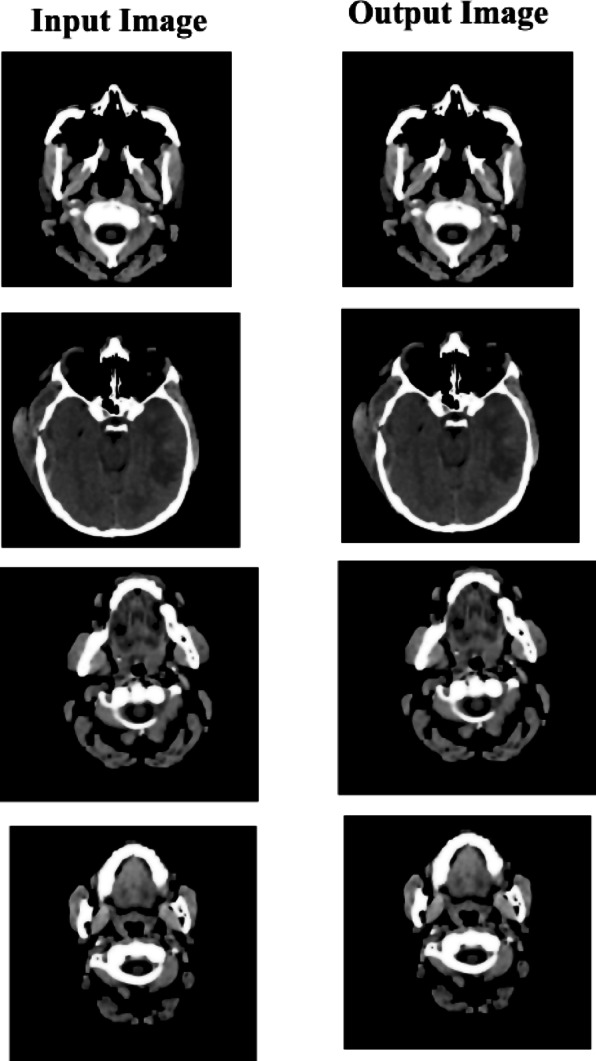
Min-max normalization rescaling CT image intensities to a common range, ensuring consistent feature scaling across federated data sources while retaining contrast critical for tumor visibility.
